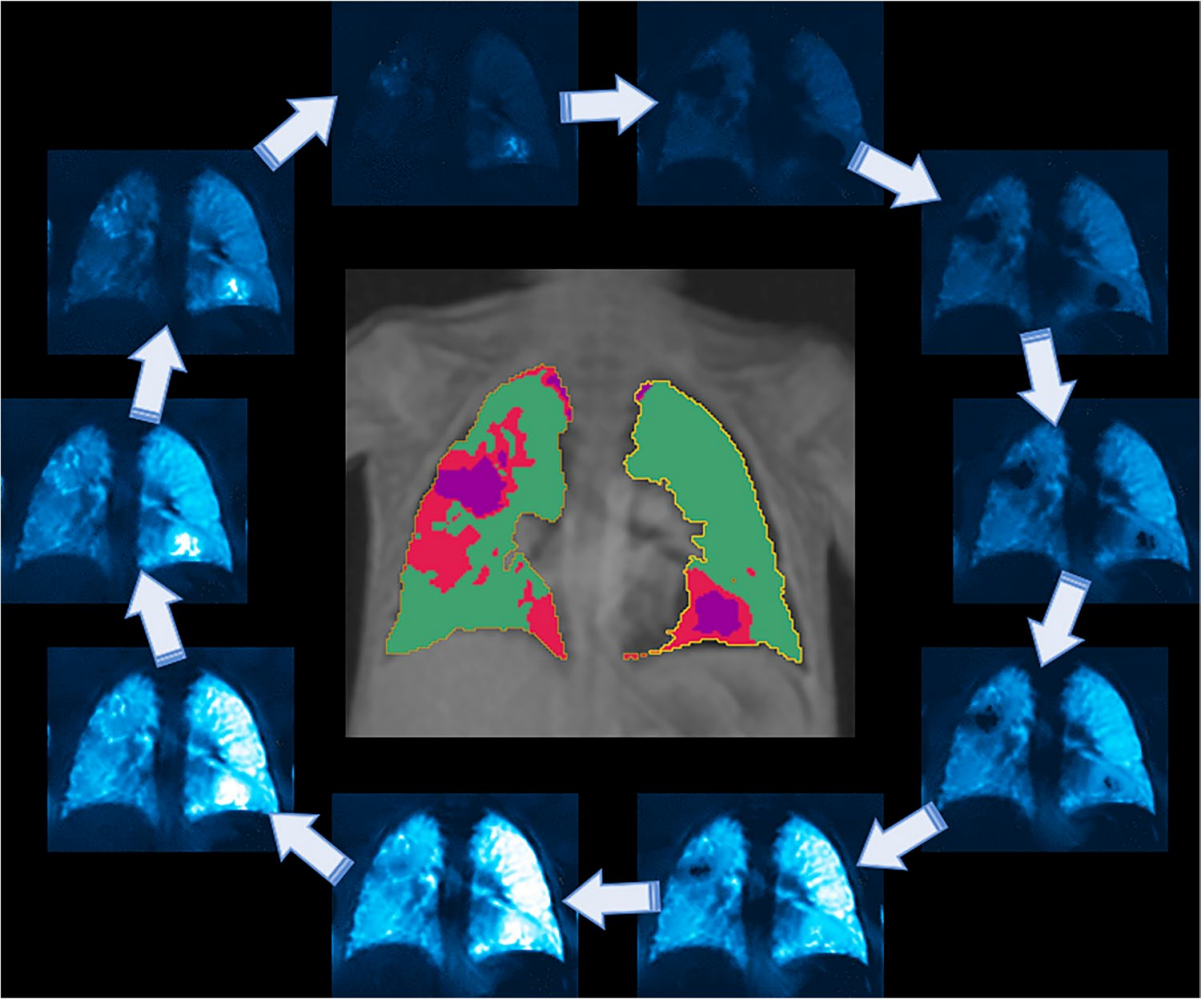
An example of an unenhanced functional proton magnetic resonance image (MRI) (Fourier decomposition) for the estimation of ventilation by means of phase-resolved functional lung MRI (PREFUL) [16] in a free-breathing 6-year-old boy after severe pneumonia (acquisition time of 60 s). Bullae can be observed in the left lower lobe (basal) and the right upper lobe, which ventilate with delayed filling and emptying of air (ventilation circle with arrows around the image). The automated computation map (within the ventilation circle) reveals healthy lung (*green*) as well as extensive damage to the lungs with perfusion deficits (*red*) and ventilation-perfusion mismatch (*purple*)
